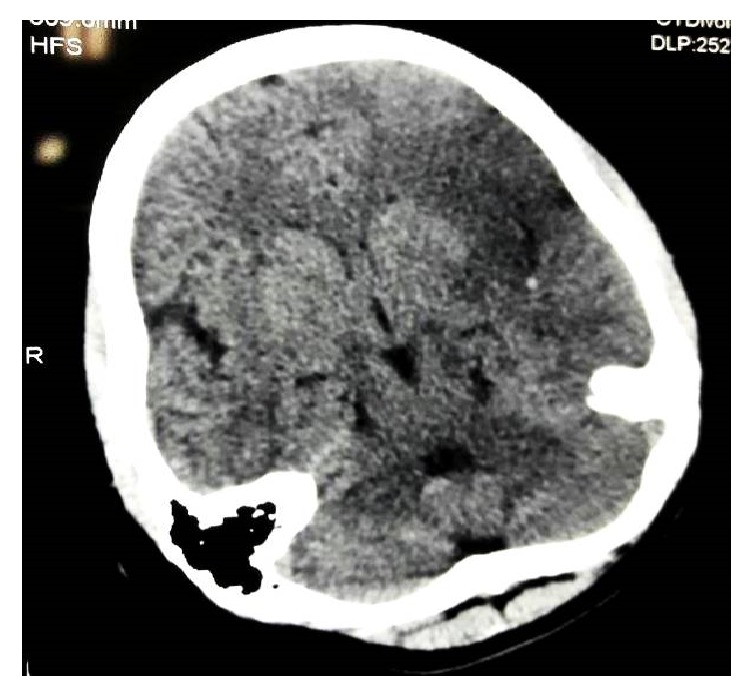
Computed tomography of head showed hypodensity at left frontotemporoparietal region along with middle cerebral artery dot sign.
